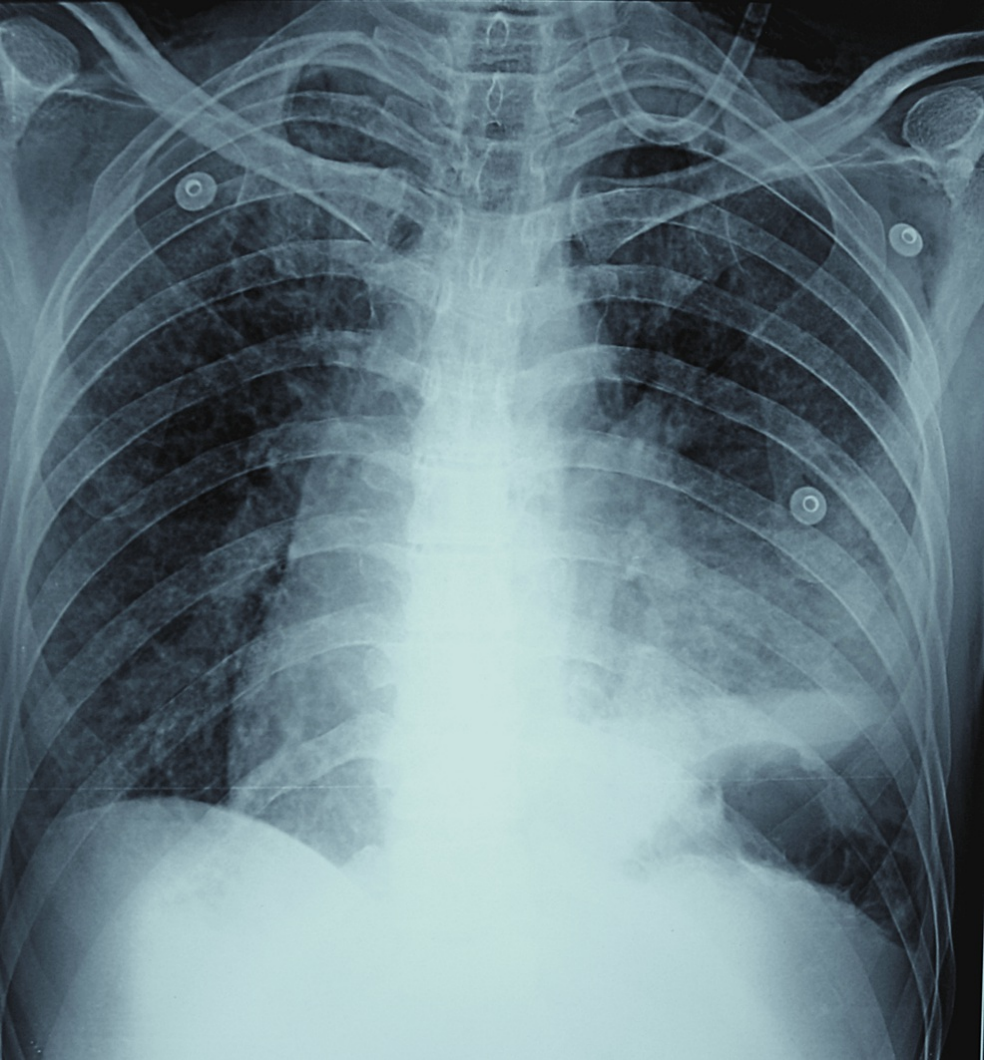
Post-tapping (thoracocentesis) chest X-ray showing reduced emphysema.
